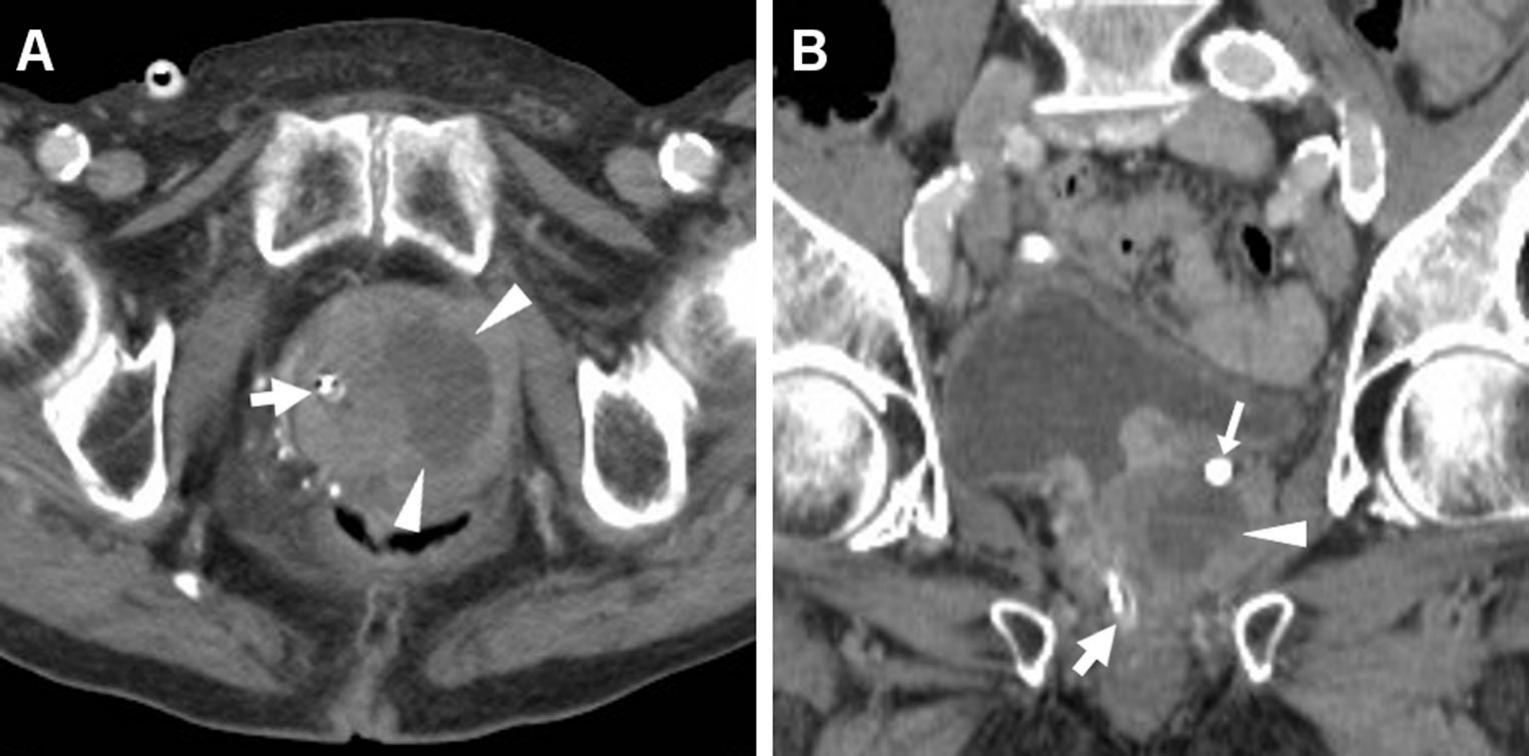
Prostate carcinoma with post-treatment regressive (necrotic) changes in an 86-year-old man with indwelling catheter (*thick arrows*). Axial (a) and coronal (b) post-contrast CT images depicted a 3×2 cm left-sided hypoenhancing region (*arrowheads*) causing enlargement of the ipsilateral prostate gland, initially interpreted as an abscess. Note residual brachytherapy seed indicated by arrow in b
